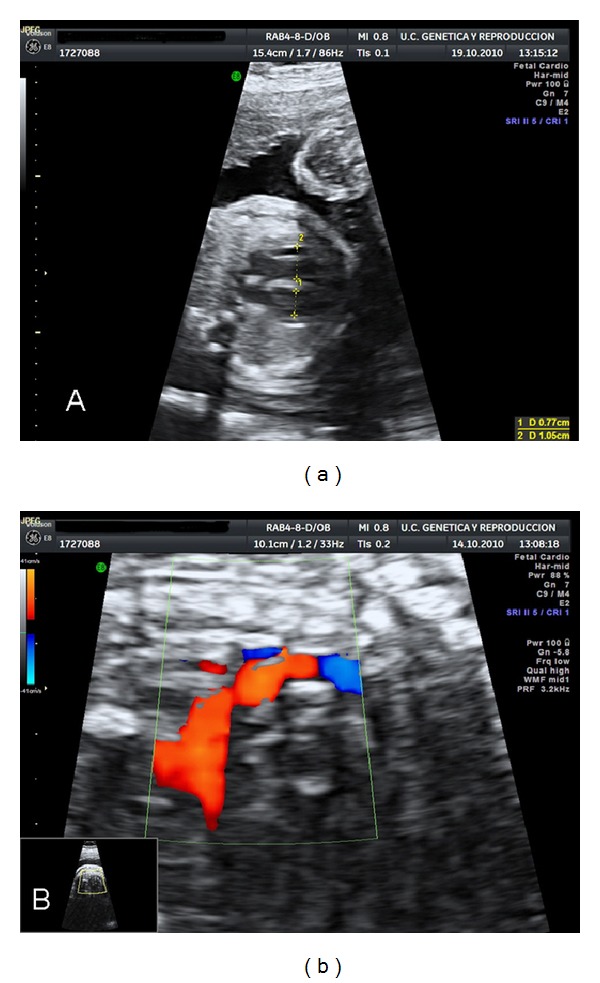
(a) Ultrasound showing chamber asymmetry. Lower: right ventricle (1.05 cm); upper: left ventricle (0.77 cm). (b) Aortic coarctation. Arrow: aortic isthmus.
